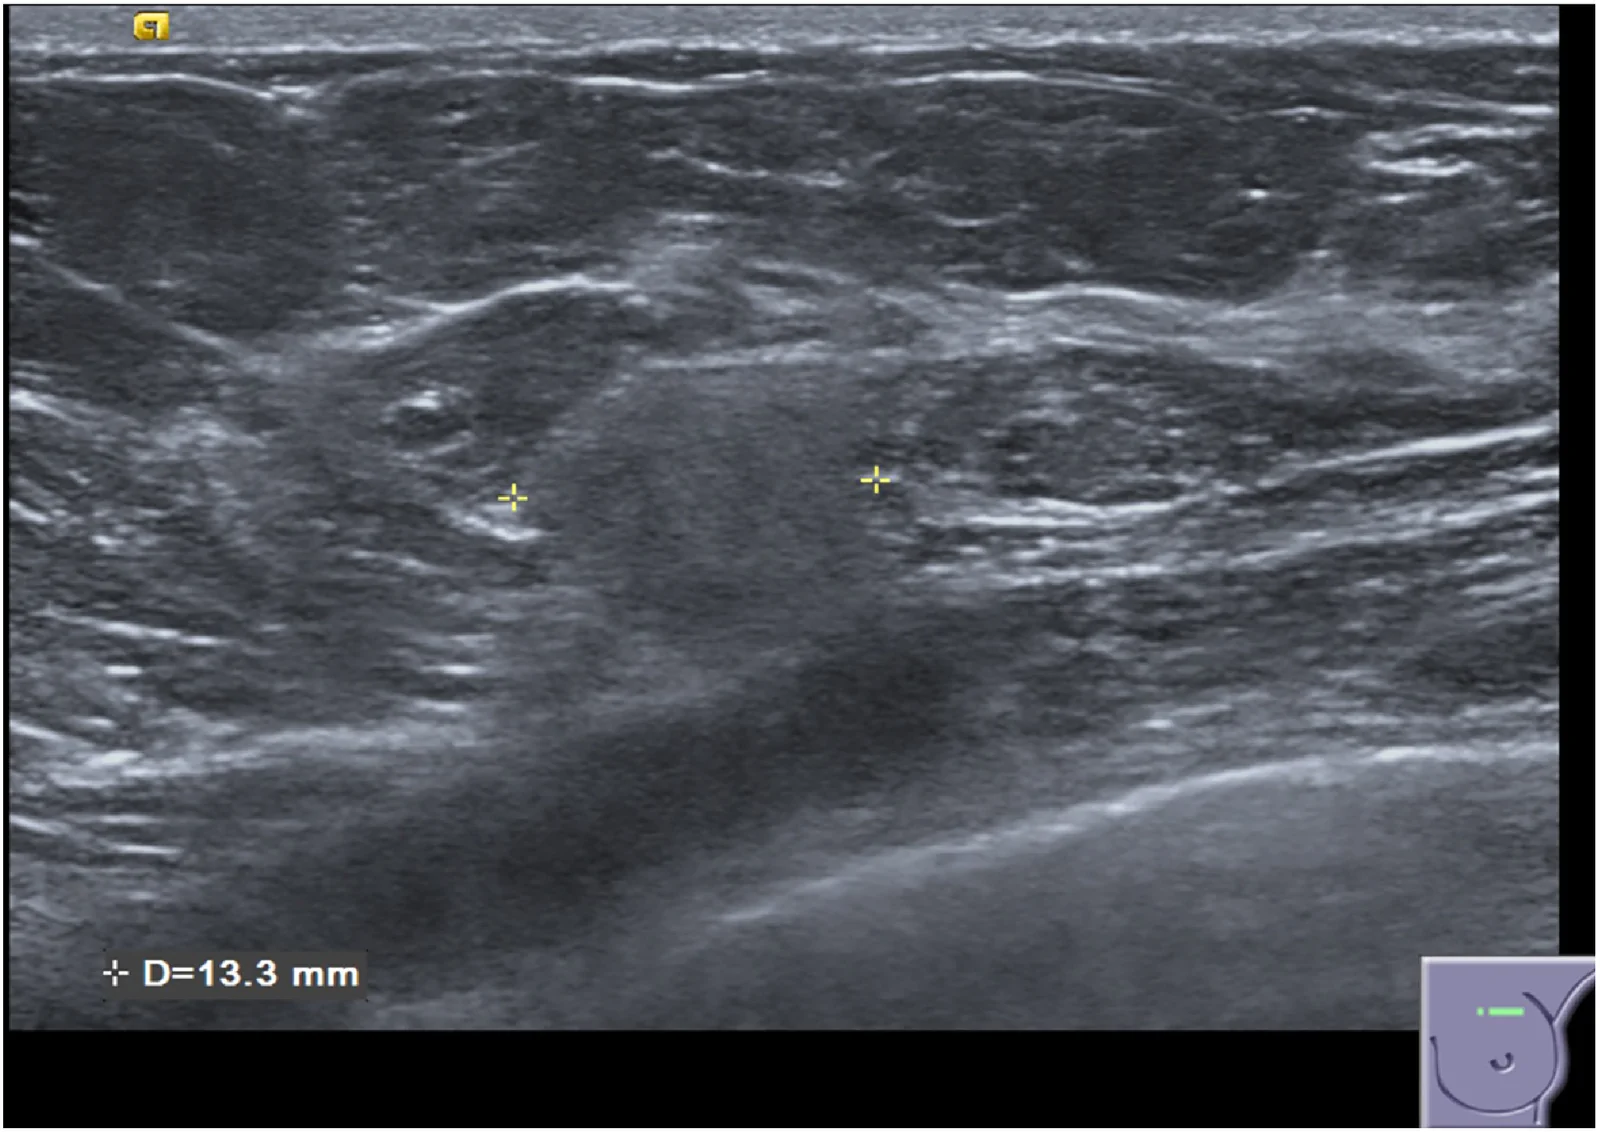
Ultrasound of the left breast demonstrates a hyperechoic 13 mm, round, partially circumscribed, partially indistinct isoechoic mass, BIRADS 4A.
